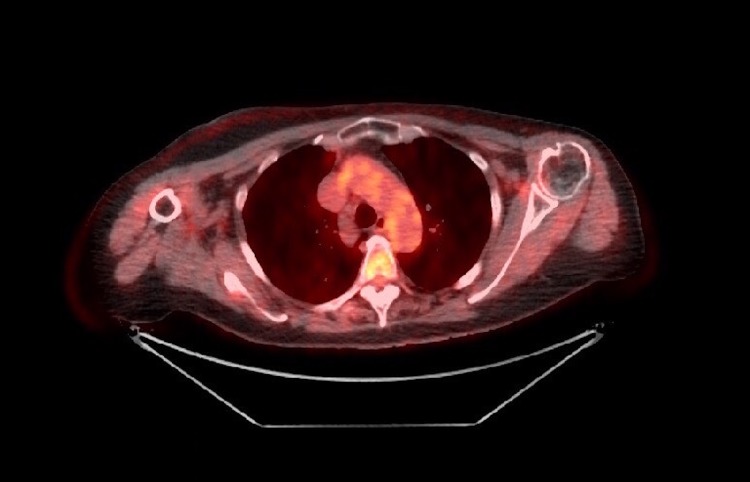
PET/CT negative for nodal disease after multimodality treatment PET/CT: positron emission tomography/computed tomography
